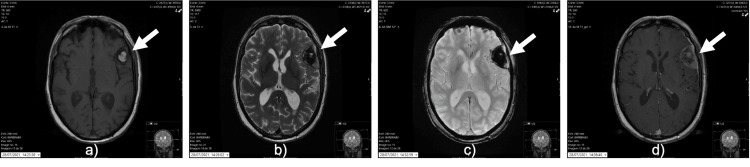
Magnetic resonance imaging at six months of follow-up showed signals of acute intratumoral hemorrhage. (a) Axial T1-weighted images showed areas of hyperintense signal inside the tumoral. (b) Axial T2-weighted MR images showed that the lesion had a heterogeneous signal. The lesion showed a marked loss of signal intensity on T2 gradient-echo images (c) and heterogeneous signal on axial T1-weighted after contrast (d).
